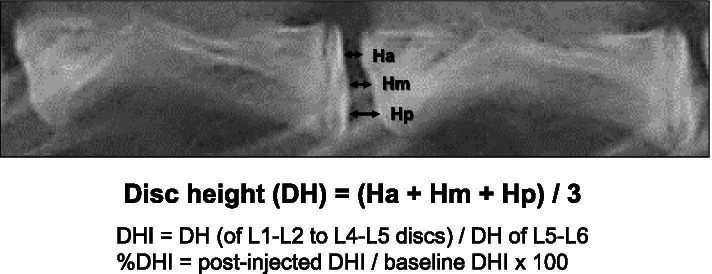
Radiographic measurement of disc height. Anterior disc height (Ha), middle disc height (Hm), and posterior disc height (Hp) were measured and calibrated. Disc height (DH) was calculated as (Ha +Hm +Hp) /3. The disc height index (DHI) was defined as: DH (L1–L2, L2–L3, L3–L4, or L4–L5 disc) / DH of L5–L6 disc (non-injection (NI) control). Change in DHI was expressed as percentage DHI (%DHI) and normalized to the measured baseline DHI: % DHI = (post-injection DHI/baseline DHI) × 100
